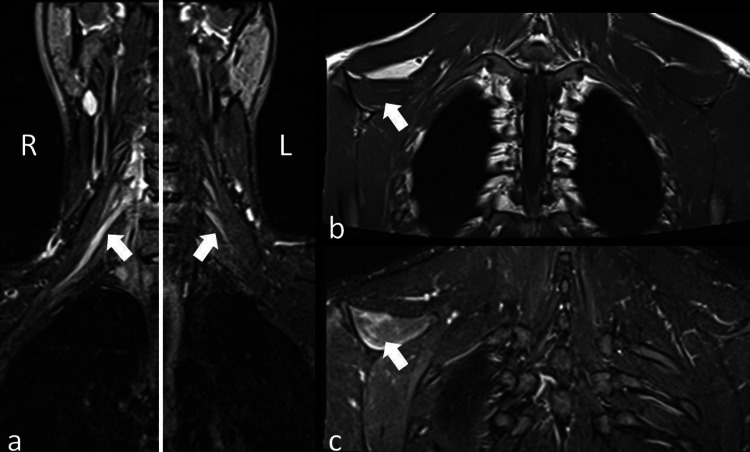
T2-TIRM MR image Coronal T2-TIRM MR image (a) with a fused view from both sides of the brachial plexus shows asymmetrical thickening of the right upper truncus (arrows). Coronal T1W image (b) shows asymmetrical volume loss (arrow) and fat-suppressed T2W image (c) shows edema (arrow) at the supraspinatus muscle indicating radiological signs of denervation atrophy. TIRM, turbo inversion recovery magnitude; MR, magnetic resonance.
